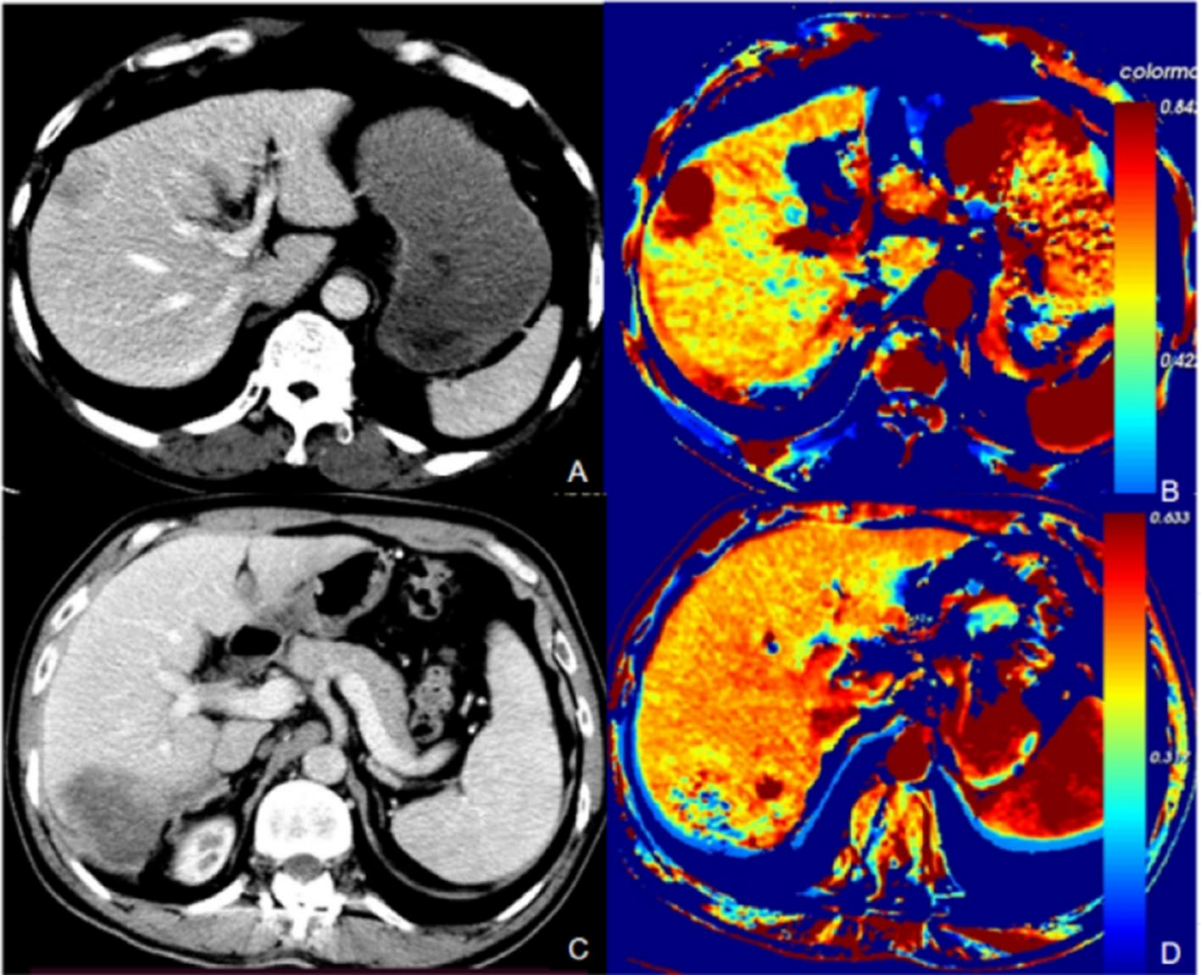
Quantitative parameter images derived from the triphasic CT scans. (A) and (C) are portal phase images of MVI negative and positive patients. (B) and (D) are corresponding perfusion images (AEF). The value of AEF in MVI negative patient was significantly higher than in MVI positive patient.
